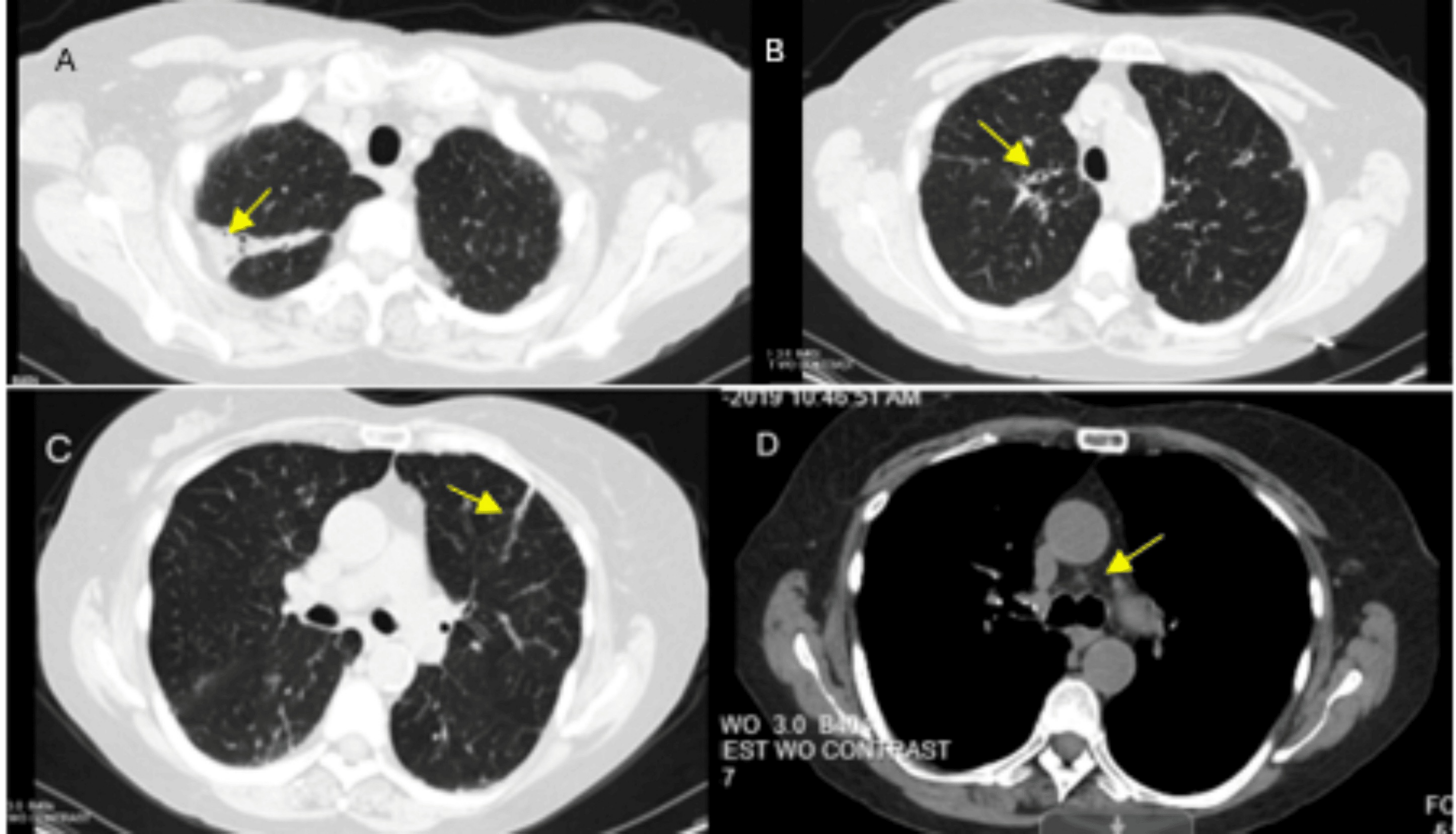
CT of the chest without contrast showing significant improvement in previously noted areas of consolidation and mediastinal lymphadenopathy (A-C). The mediastinal window reveals significantly improved right paratracheal lymphadenopathy (D).
